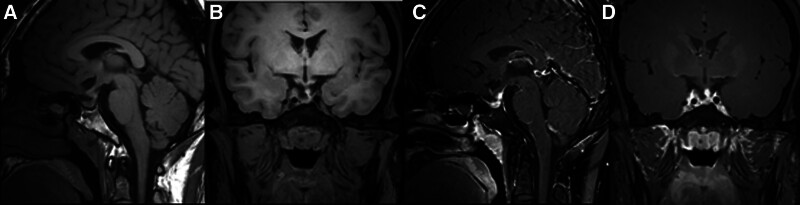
Patient MRI on June 27, 2021 (A and B: T1WI; C and D: enhanced MRI).
